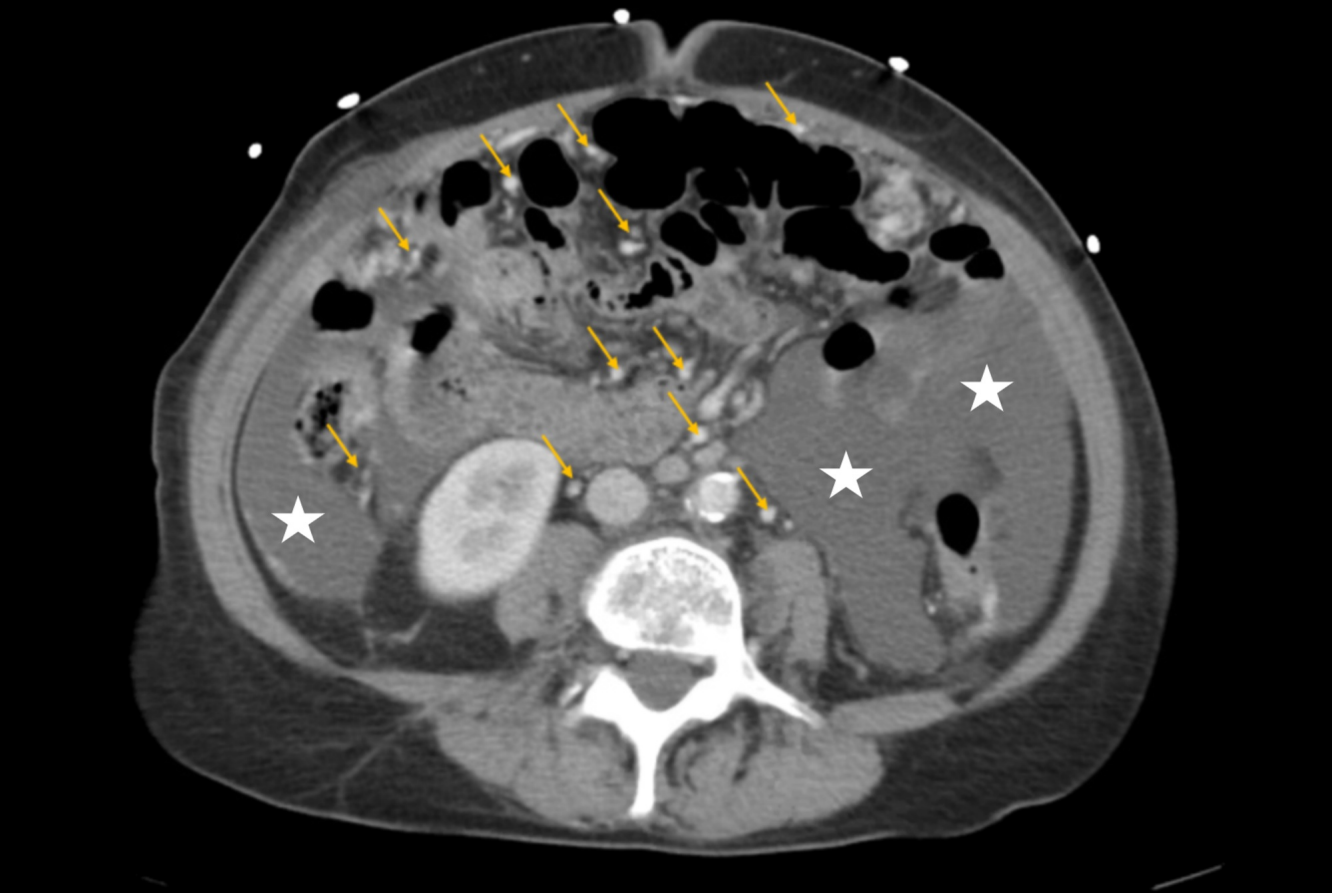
CT scan of the abdomen and pelvis showing ascites (white stars) with mesenteric, and retroperitoneal lymphadenopathy (yellow arrows)
